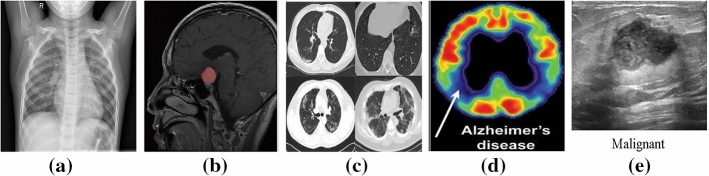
Samples of a a normal chest from the Chest X-Ray Images dataset (Kermany et al. 2018), b a brain with meningioma MRI Image, retrieved from the Brain MRI Images for Brain Tumor dataset (Cheng 2017), c CT scans for COVID-19 patients from the COVID-CT-Dataset (Zhao et al. 2020), d PET scans from TADPOLE challenge PET data (Marinescu et al. 2018) for an Alzheimer’s disease and e an image from the Ultrasound breast images dataset (Al-Dhabyani et al. 2020)
